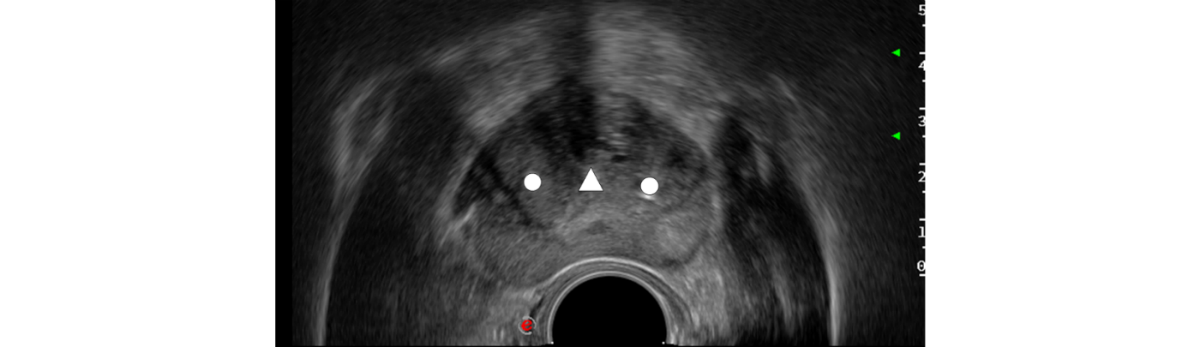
Transverse plane ultrasound image of the prostate. Fibres (circles) positioned in both transition zones while maintaining a safe distance from the urethra (triangle), rectum and prostatic capsule.
